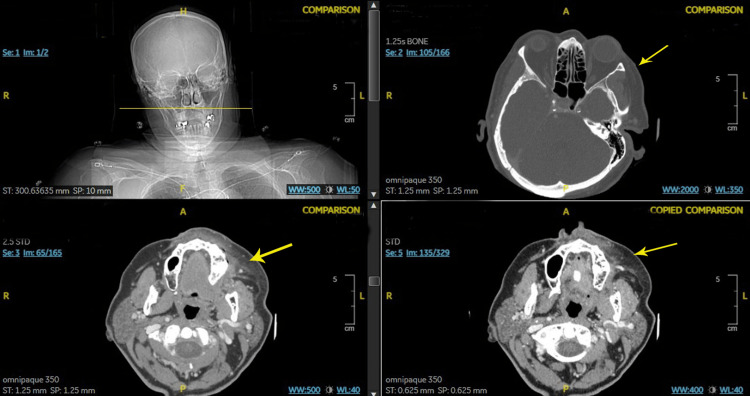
CT scan of the facial bones showing edema and stranding within the soft tissues and subcutaneous fat superficial to the left parasymphyseal mandible and maxilla (yellow arrows). CT: computed tomography
